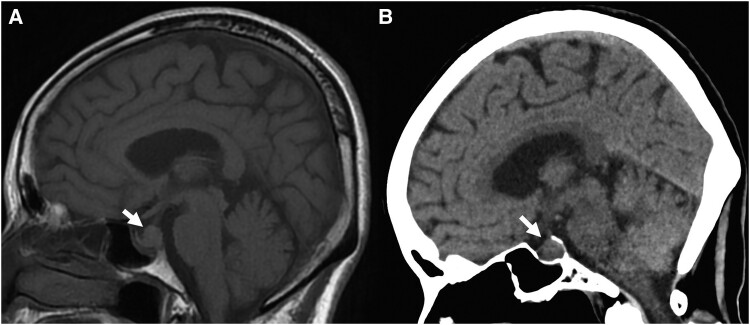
Sagittal images of the head on A, magnetic resonance imaging and B, computed tomography at the initial presentation, showing an enlarged pituitary gland with the absence of the posterior pituitary bright spot and no calcification (arrows).
